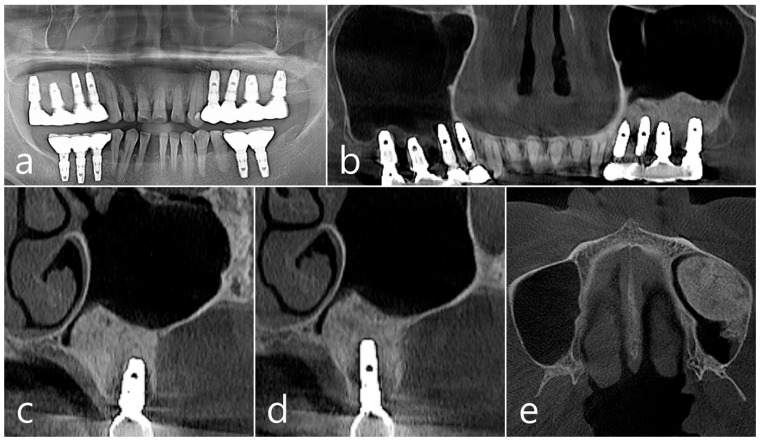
(a,b) In the panoramic radiography and CBCT images taken after the prosthesis was delivered, no special radiographic image was observed at the left sinus augmented site; (c,d) In coronal images of CBCT scanned in #26 and #25 implants, a homogenous radiographic density was observed at the sinus augmented site, and there was no thickening of the sinus mucosa; (e) A well-augmented sinus graft was observed in CBCT axial image.
